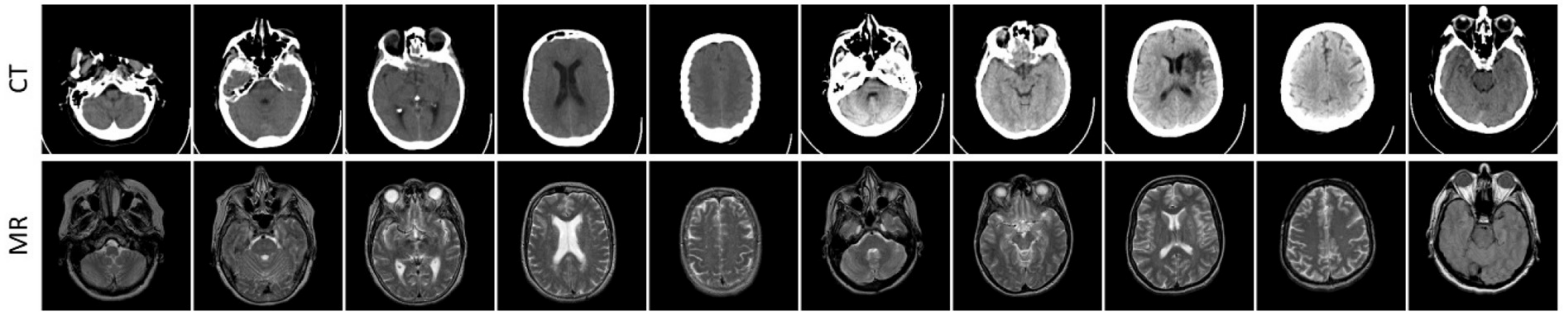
Ten pairs of images in the CT-MR image dataset.
